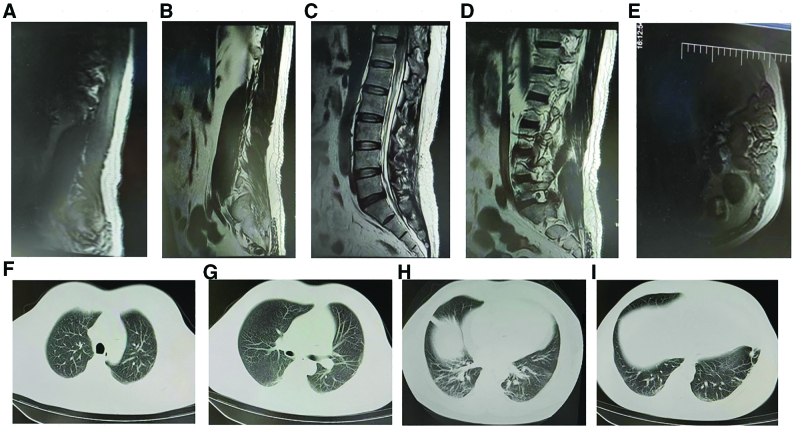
Changes in chest computed tomography (CT) and magnetic resonance imaging (MRI) of the thoracic and lumbar spine after 3 wk of treatment. (A–E) Changes in the thoracic and lumbar spine MRI after 3 wk of treatment. (F–I) Changes in the chest CT after 3 wk of treatment.
